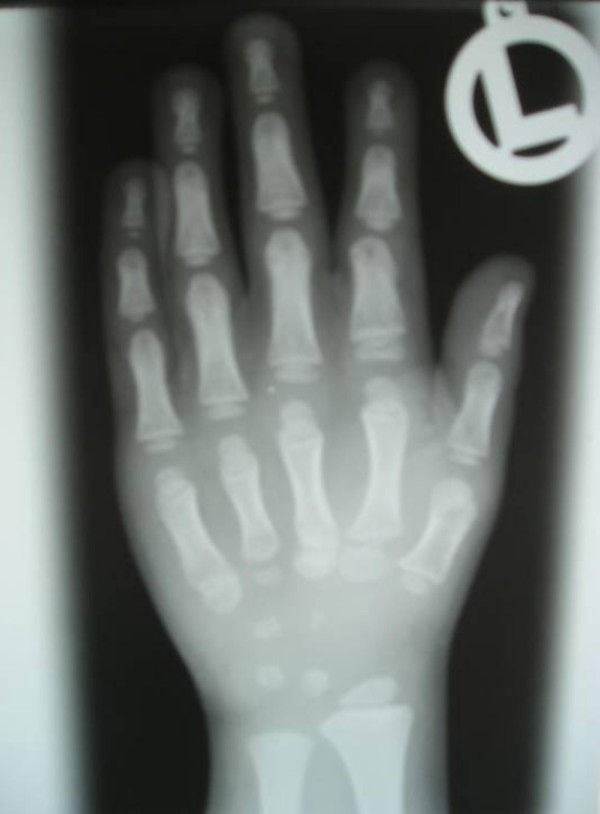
Anteroposterior hand radiograph showed defective ossification of the carpal bones, the proximal phalanges of the first and fourth fingers were short and broad.
